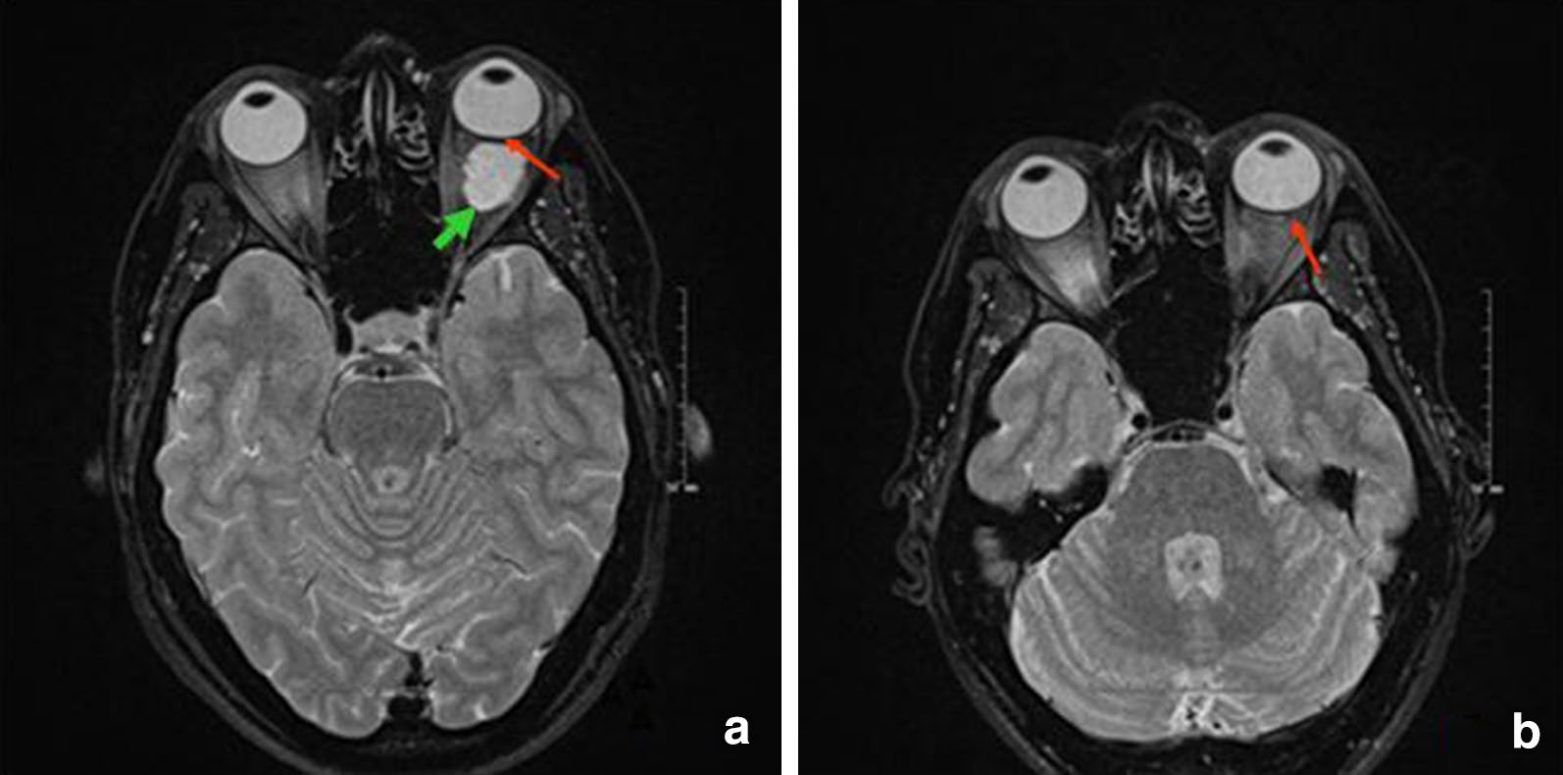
Magnetic resonance imaging of the orbit. Magnetic resonance imaging (T2), axial view showing the patient at presentation (a) with intraconal mass in the left orbit (*green arrow*), and flattening of the posterior pole (*red arrow*); 3 years after complete removal of the tumour, posterior pole flattening (*red arrow*) persisted (b)
